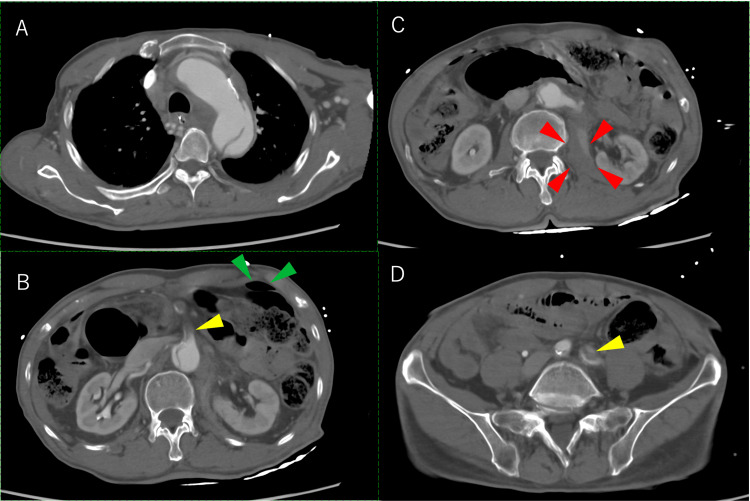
Contrast-enhanced CT after intubation (A) Contrast-enhanced CT after intubation showing aortic dissection and disruption of blood flow to the CA, SMA (B, yellow arrowheads), and left common iliac artery (C, red arrowheads). Free air was observed in the abdominal cavity (B, green arrowheads) and retroperitoneal hematoma (D, yellow arrowheads). CA, celiac artery; CT, computed tomography; SMA, superior mesenteric artery
